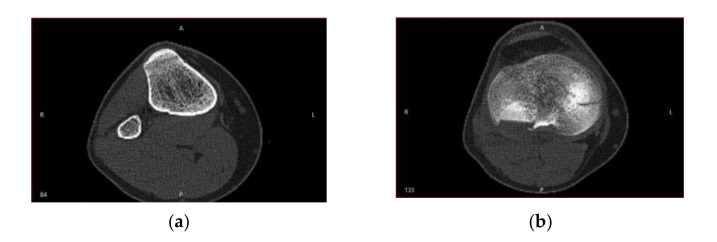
Cortical and cancellous tissue view in a CT: (a) high-intensity circular regions show thick cortical bone tissue in the median area of the tibia; (b) more proximal, there is much less contrast between the regions of cortical and cancellous bone tissue.
